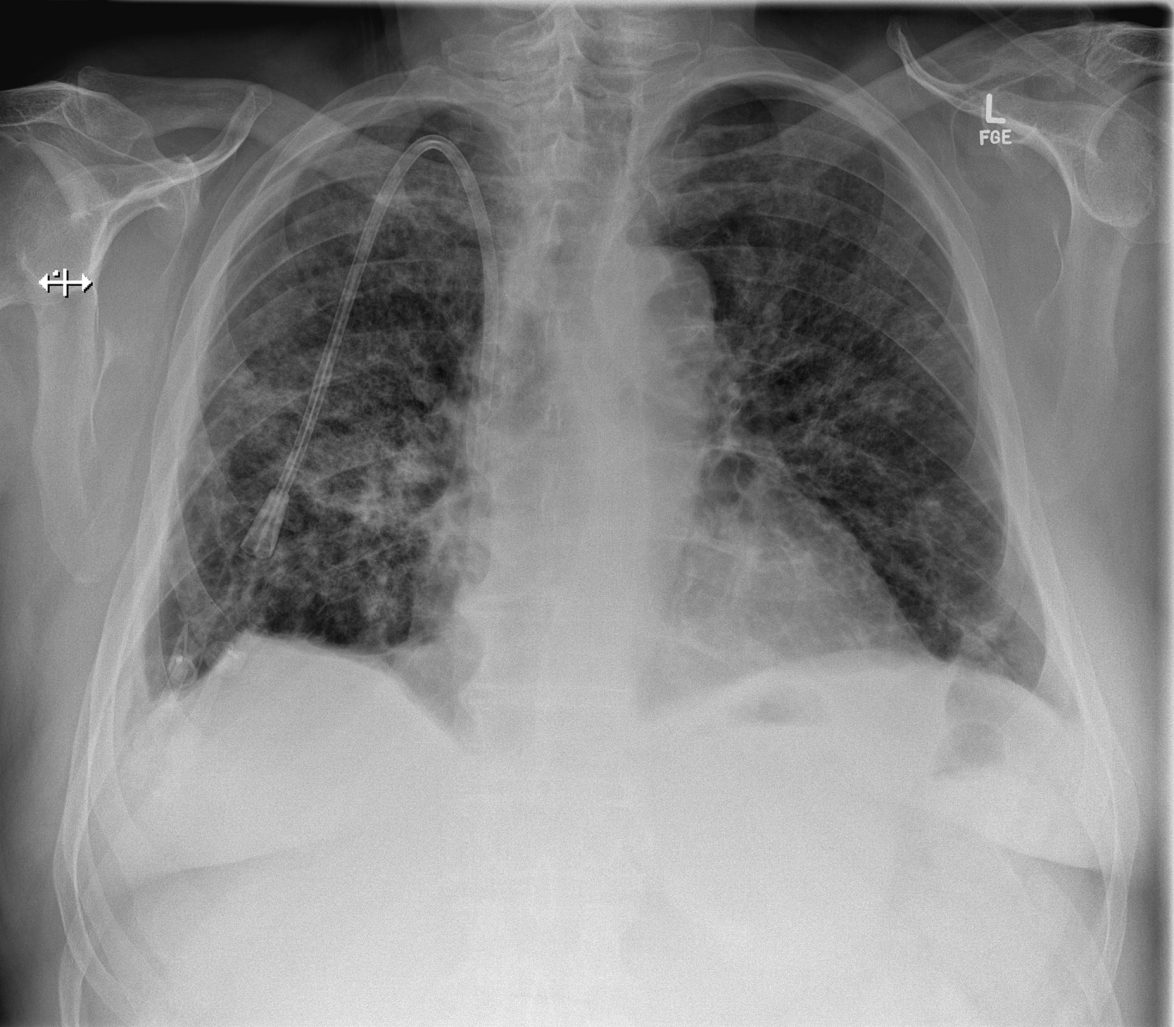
Chest radiograph at time of discharge. Significant improvement in bilateral infiltrates.
